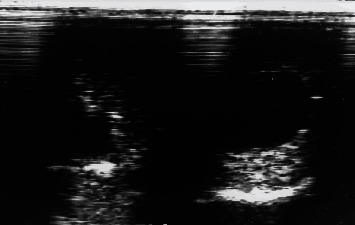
Ultrasound image of bilateral intratesticular spermatoceles in a Dama gazelle. Spermatocele appears as fluid-filled (anechoic) cystic structures.
